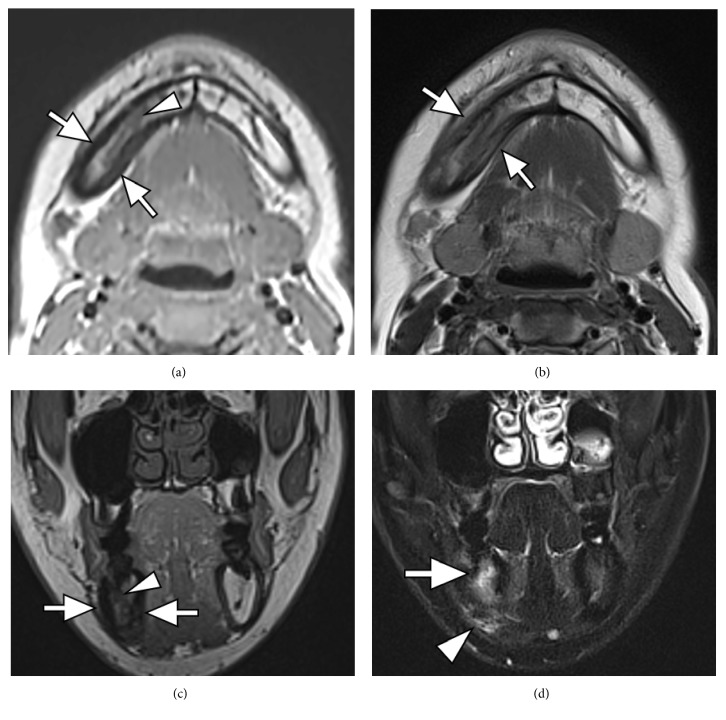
MR imaging of the mandible. Axial (a) and coronal (c) T1-weighted images and axial T2-weighted image (b) show thickening of the right mandible (arrows) and decreased bone marrow fat signal (arrowheads). Coronal short tau inversion recovery image (d) shows high signal intensity in the right body of the mandible (arrow), with perilesional soft tissue swelling (arrowhead).
